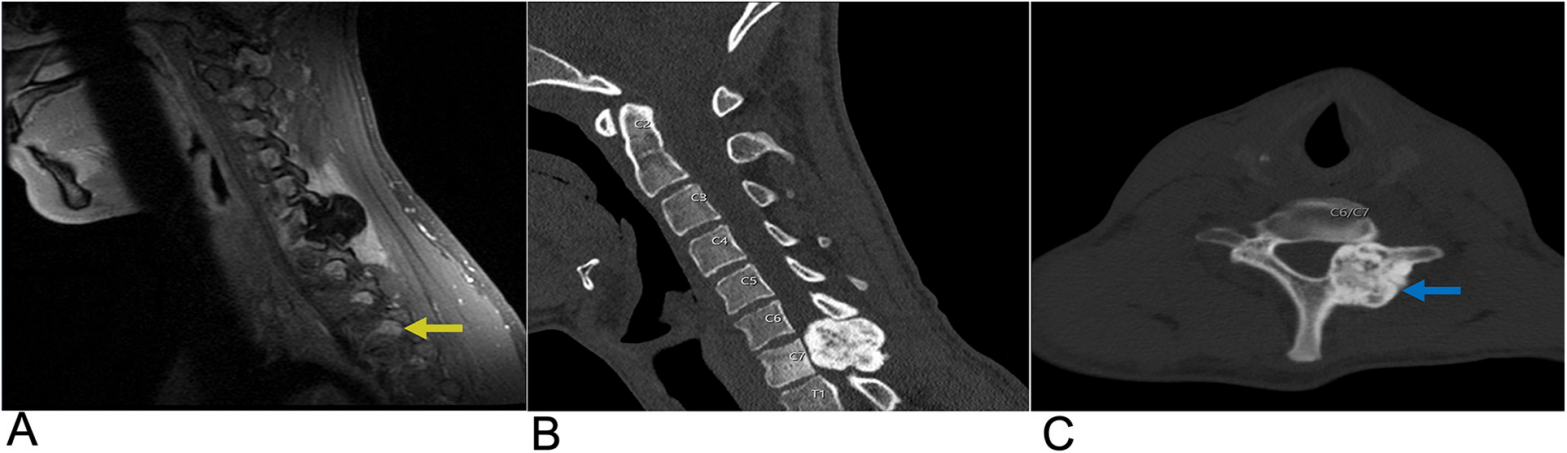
Osteoblastoma. Sagittal T1 + C MRI (A) as well as Sagittal CT (B) and Axial CT (C) of the cervical spine demonstrating a calcified enhancing lesion (yellow arrow) in the C7 left lateral mass representing osteoblastoma (blue arrow).
